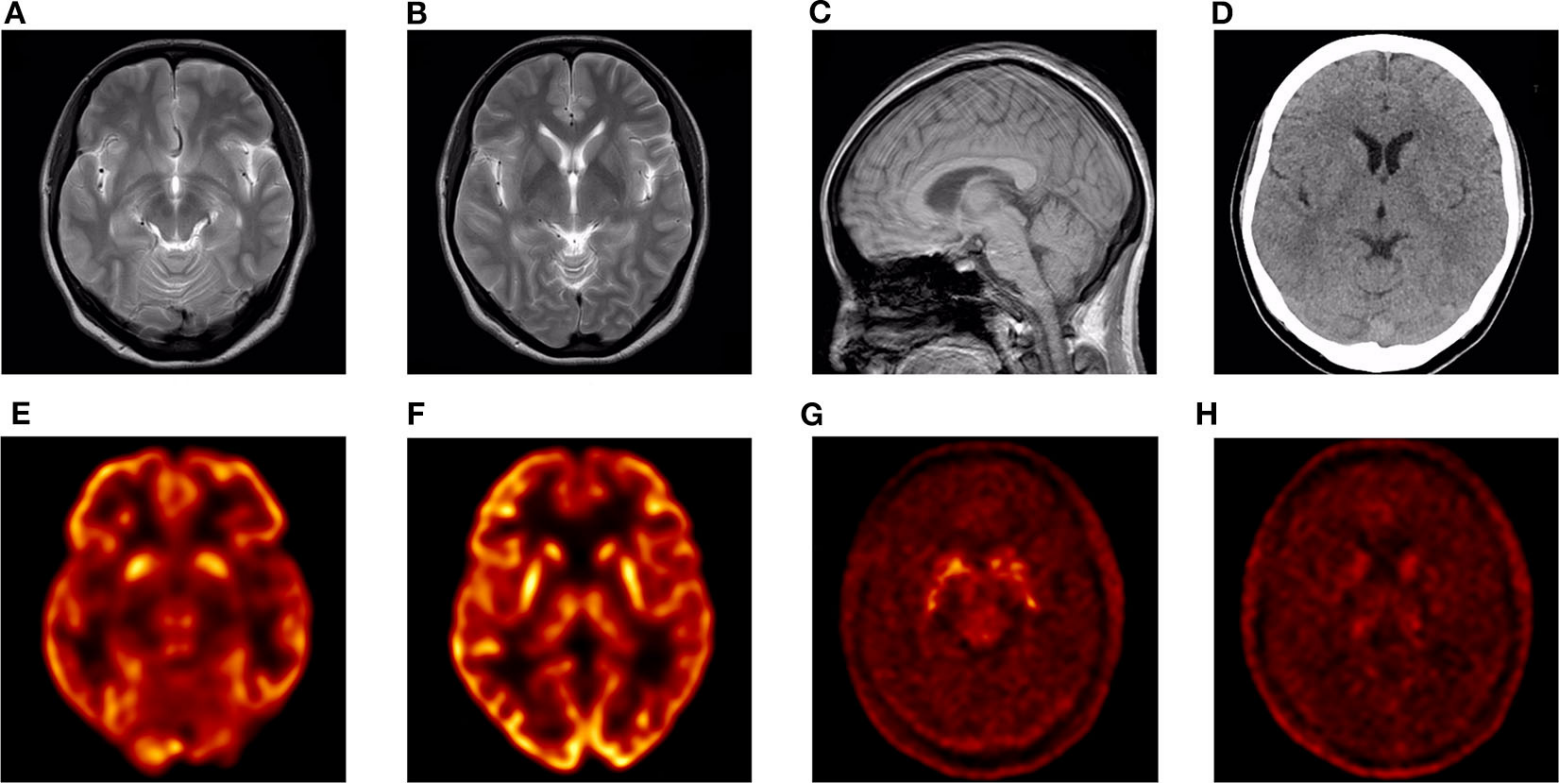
Neuroimaging images of the proband. (A–C) 3.0T MRI images: no obvious abnormalities. (D) CT image: no obvious abnormalities. (E,F)
